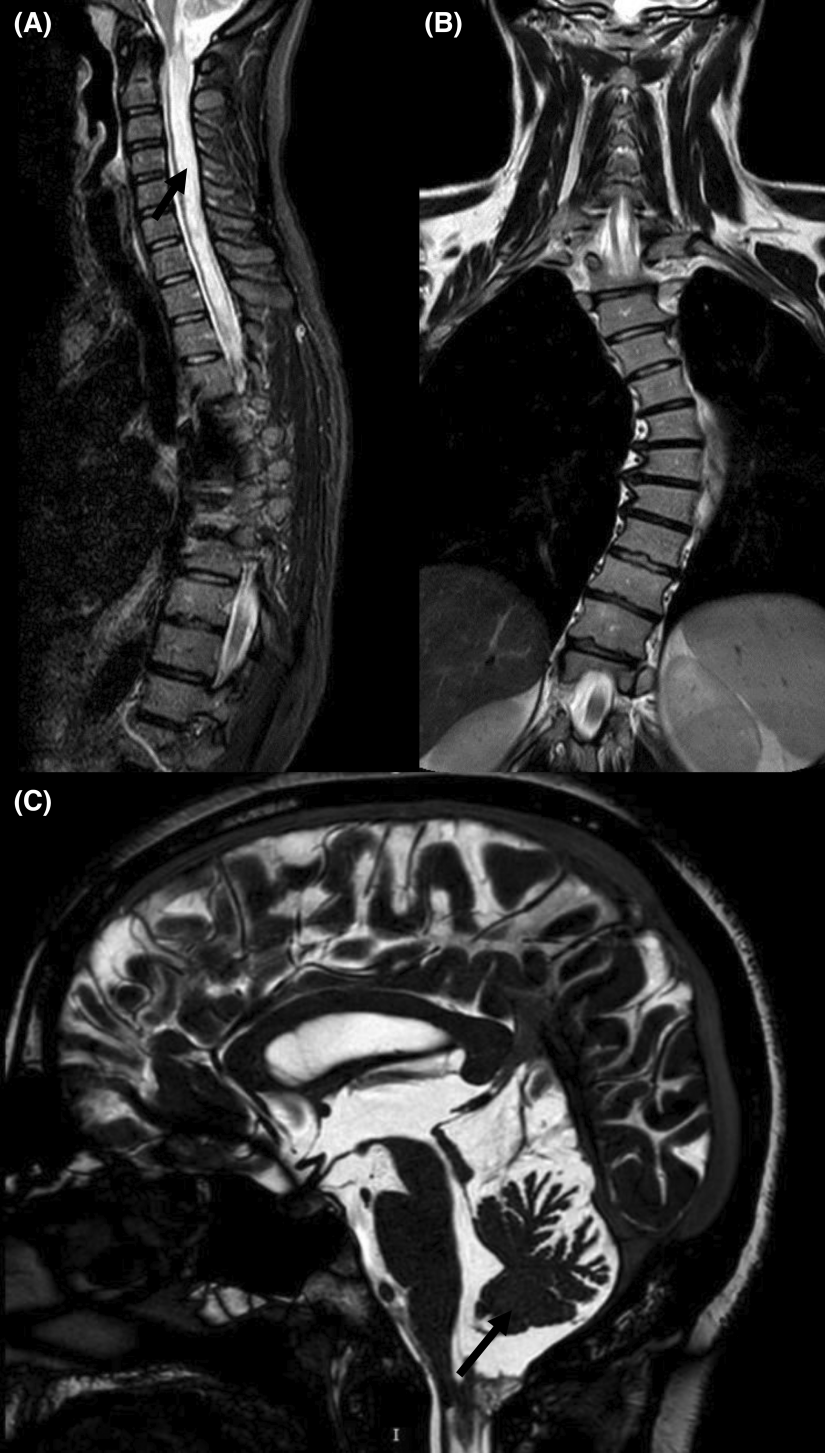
Sagittal T2‐weighted MRI of the cervicothoracic spine demonstrating a large holocord syrinx (arrow) extending from C2 to the conus medullaris (A); coronal T2‐weighted MRI of thecervicothoracic spine demonstrating a large S‐shape scoliosis with lower curve at L1 with convexity to right and upper curve at T6 with convexity to left (B); sagittal T2‐wighted MR images of the brain demonstrating severe adhesive arachnoiditis (arrow) and CSF blockade in craniocertebral junction (CVJ) (C).
